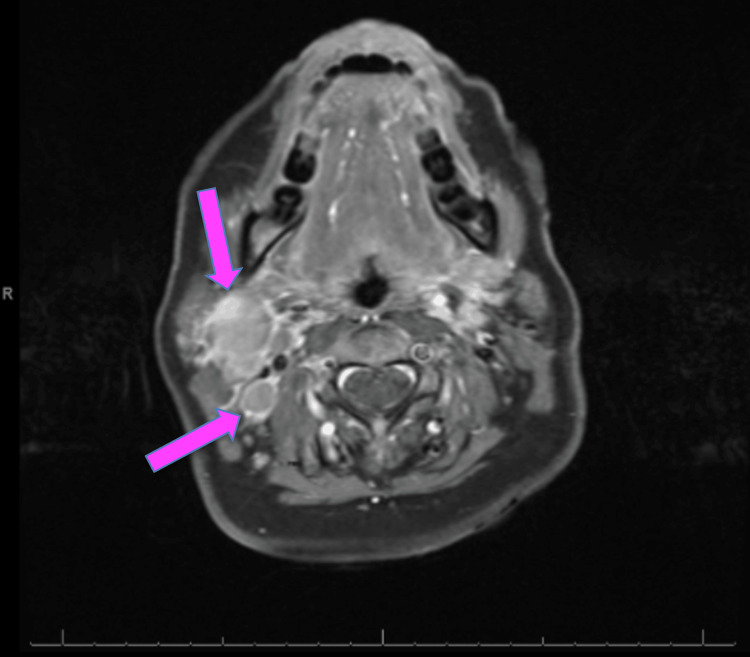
MRI of the neck revealed multiple right side lymph nodes, the largest being 2.3 cm
